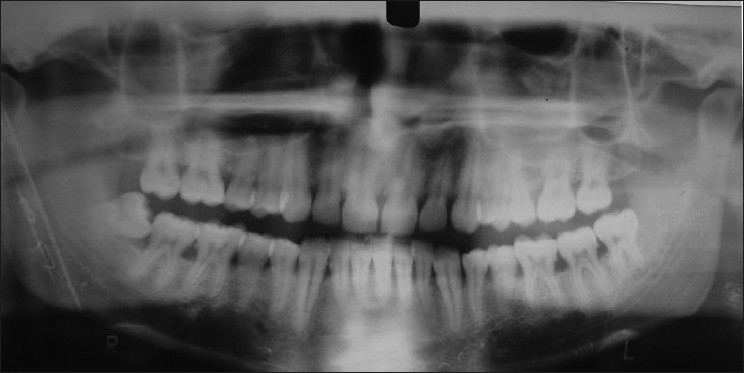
Orthopantomograph of subject showing 5 mandibularincisors and bone loss
